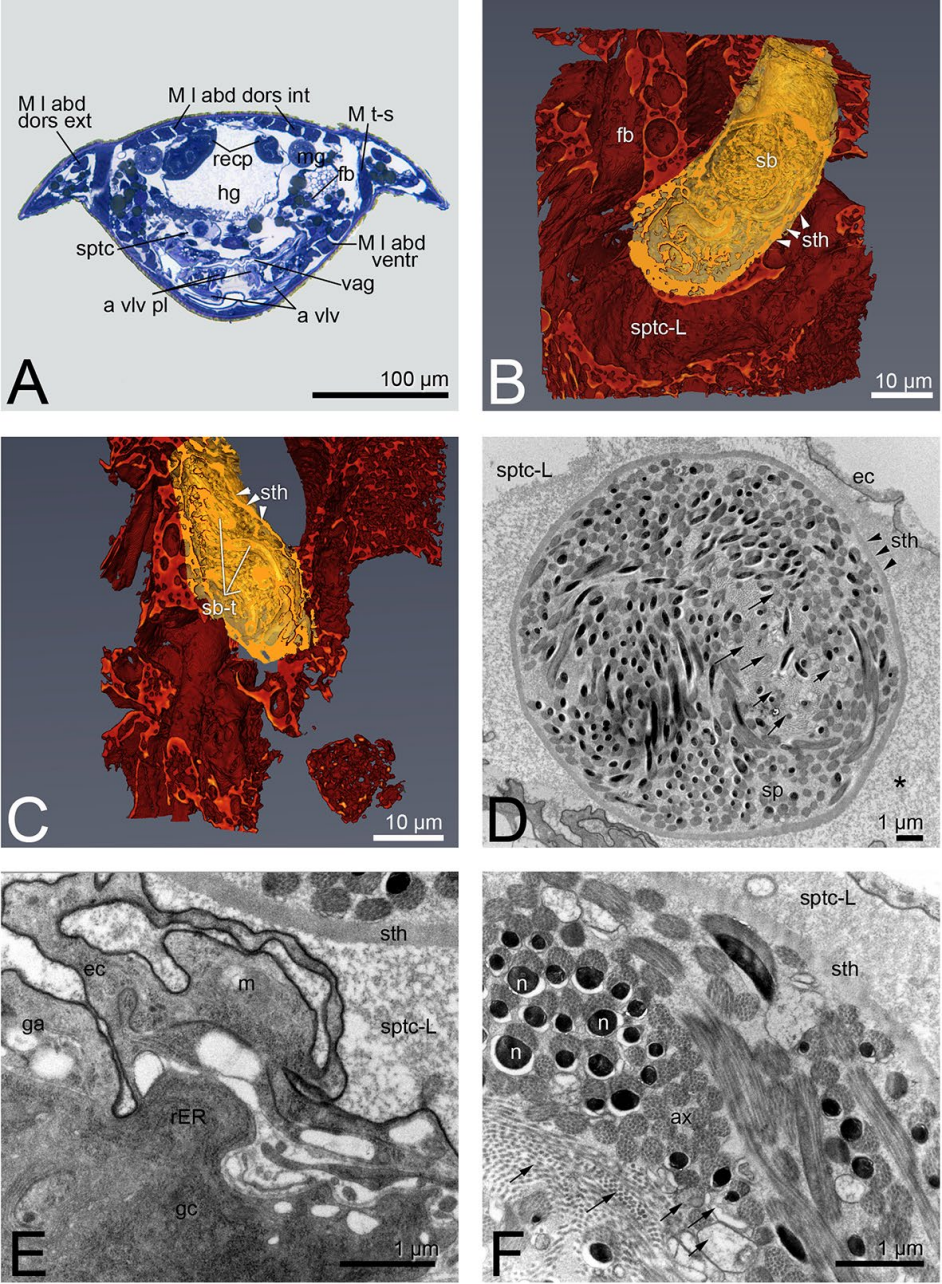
Hercinothrips femoralis*, (A) location relationship of the spermatheca within the body cavity, transverse section; (B) spermatheca filled with a sperm ball of compressed secretion and spermatozoa, nano CT; (C) spermatheca filled with a sperm ball with several tubes; (D) sperm ball within the secretion-filled spermatheca; (E): epithelial layer of the spermatheca; (F) ultrastructure of defective and intact spermatozoa within the sperm ball.
